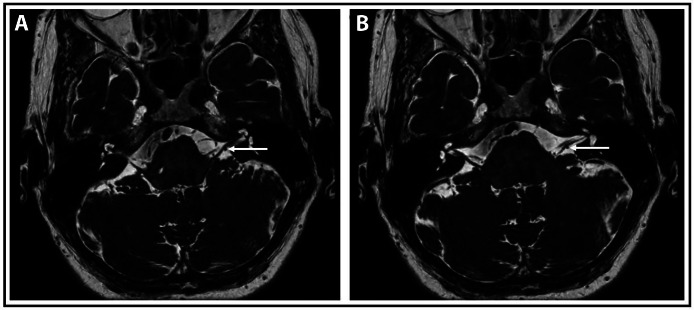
High-resolution MRI demonstrating neurovascular compression of the left vestibular nerve. (A and B) Heavily T2-weighted CISS/FIESTA images show close contact between the anterior inferior cerebellar artery (AICA) and the vestibulocochlear nerve within the cerebellopontine angle cistern (arrows). These high-resolution sequences provide excellent contrast between cerebrospinal fluid, cranial nerves, and vascular structures, facilitating identification of neurovascular conflict consistent with vestibular paroxysmia.
